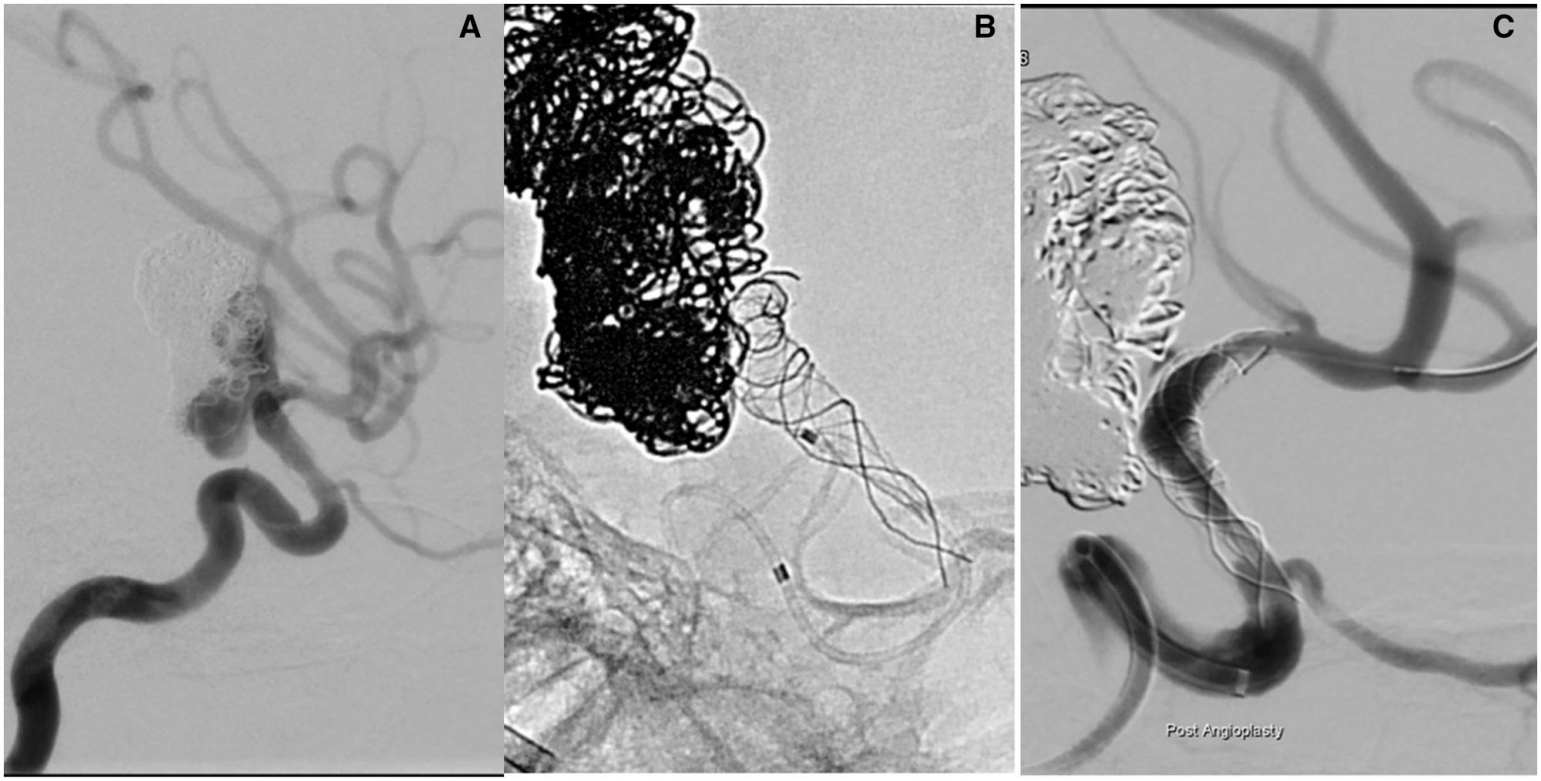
(A) An additional 6 months later, the boy returned for further treatment as an MRI had shown aneurysm recurrence. The diagnostic angiogram was thought to show resolution of the intimal changes. It was possible to advance a microcatheter into the left M1 without problems. (B) Deployment of the SILK flow-diverting stent. The proximal end of the stent does not open. The ICA occludes and it is not possible to advance the microcatheter over the stent delivery wire. (C) After angioplasty with a Maverick coronary balloon flow is restored after 5 h. There remains a significant residual stenosis. Abbreviation: ICA = internal carotid artery.
